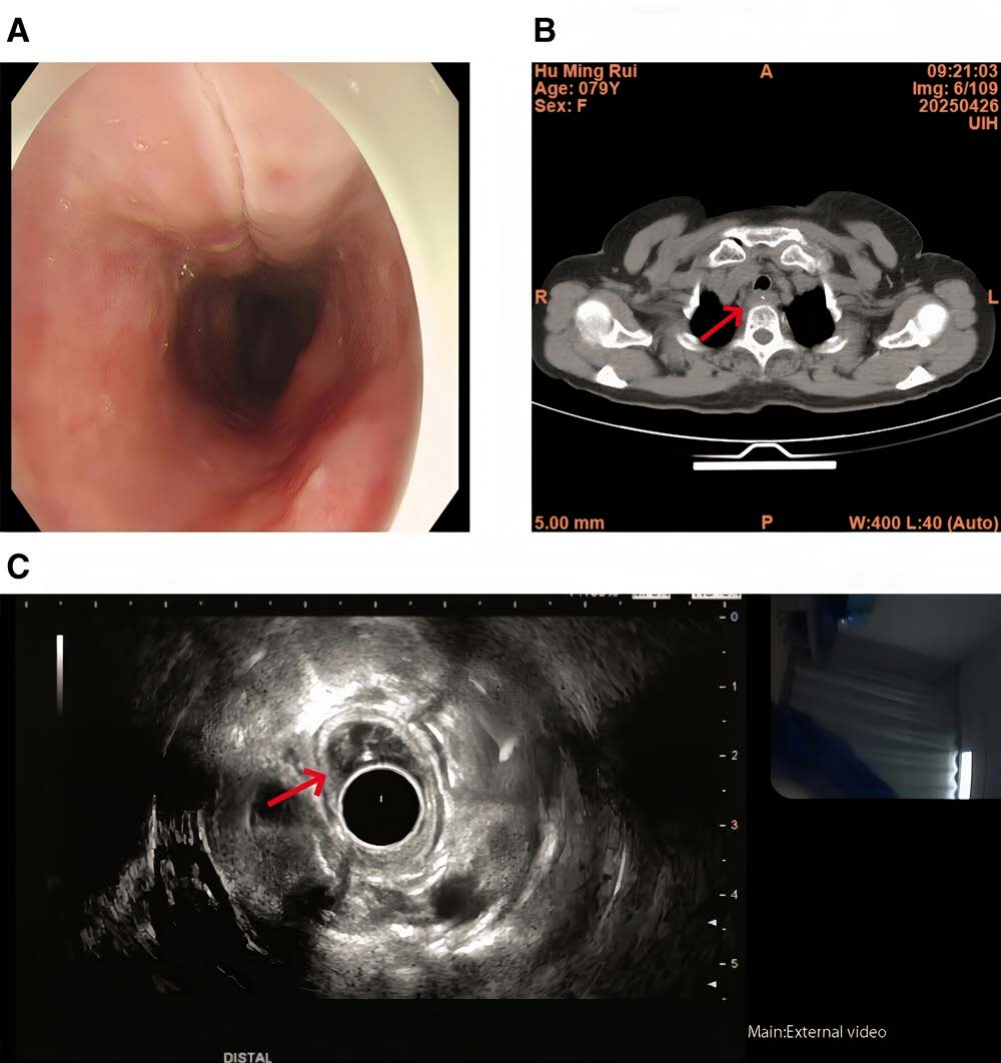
(A) Esophagogastroduodenoscopy finding. (B) Cervical/thoracic CT revealed a hyperdense shadow in the proximal esophagus, with no radiographic signs of perforation. (C) Endoscopic ultrasound (EUS) identified a 5.4 mm thick heterogeneous hypoechoic area containing a hyperechoic core. CT = computed tomography.
